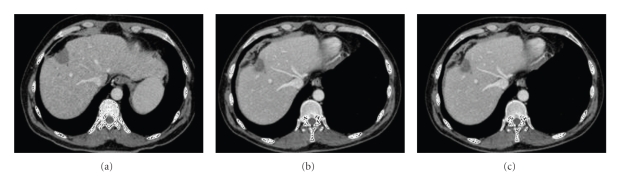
CT scans 15 days (a), 6 months (b), and 20 months (c) after liver resection for HCC in cirrhotic liver.
